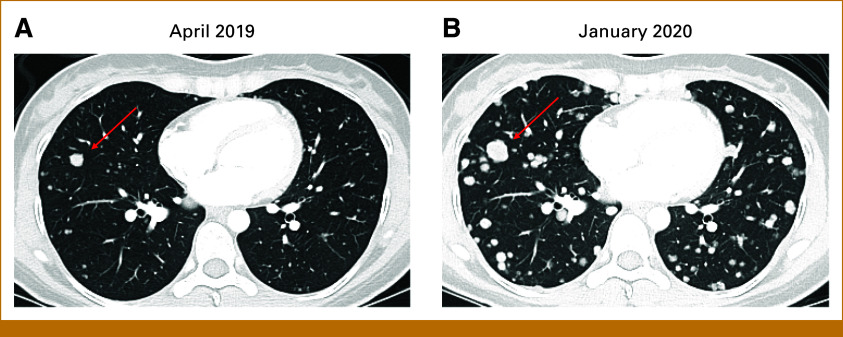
Chest CT shows progression of ACC lung metastases and the development of pleural lesions while on active surveillance over a period of 9 months. (A) April 2019: multiple bilateral nodules, with the largest measuring 10.5 mm (arrow). (B) January 2020: progressive growth of existing lesions and appearance of multiple new lesions and nodular pleural thickening (not shown). The previous largest lesion grew from 10.5 mm to 16.7 mm (arrow), representing an increase of 59%. ACC, adenoid cystic carcinoma; CT, computed tomography.
